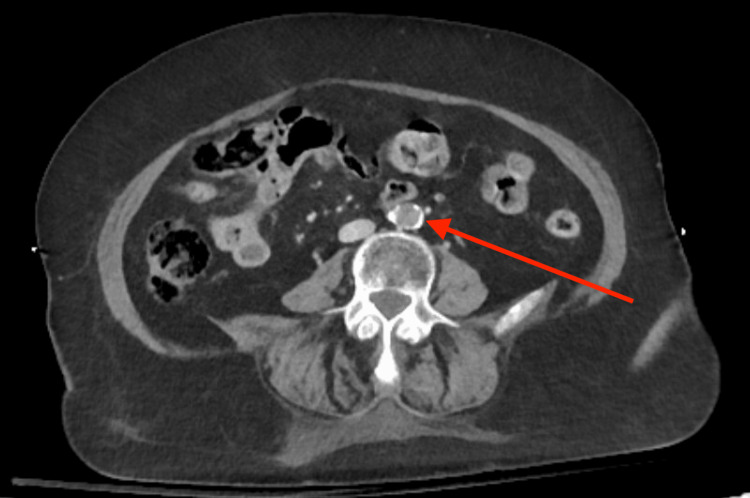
Computed tomography with angiography of the abdomen and pelvis on axial slice demonstrating the distal aorta occluded just proximal to the bifurcation. The red arrow denotes a 3-cm distal aortic plaque/thrombus.
